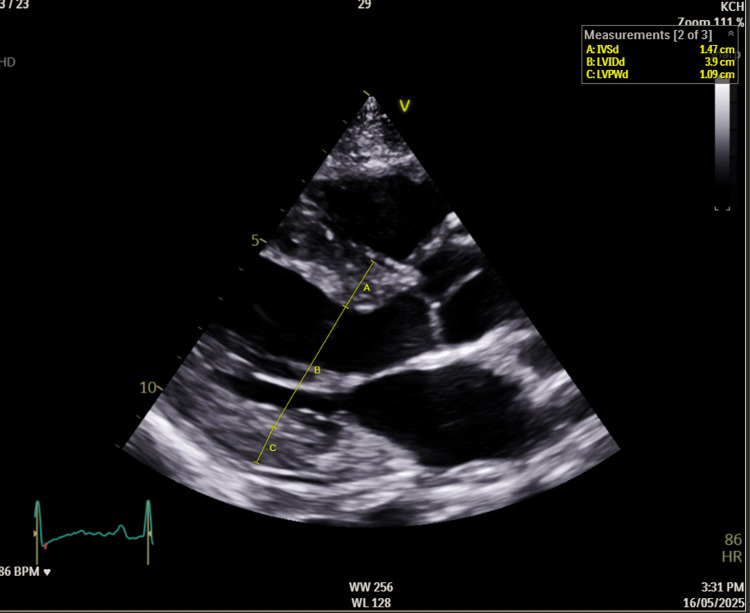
Parasternal long axis view with measurement of IVSD, LVIDD, and LVPWD IVSD: interventricular septal diameter; LVIDD: left ventricular internal diameter; LVPWD: left ventricular posterior wall diameter
